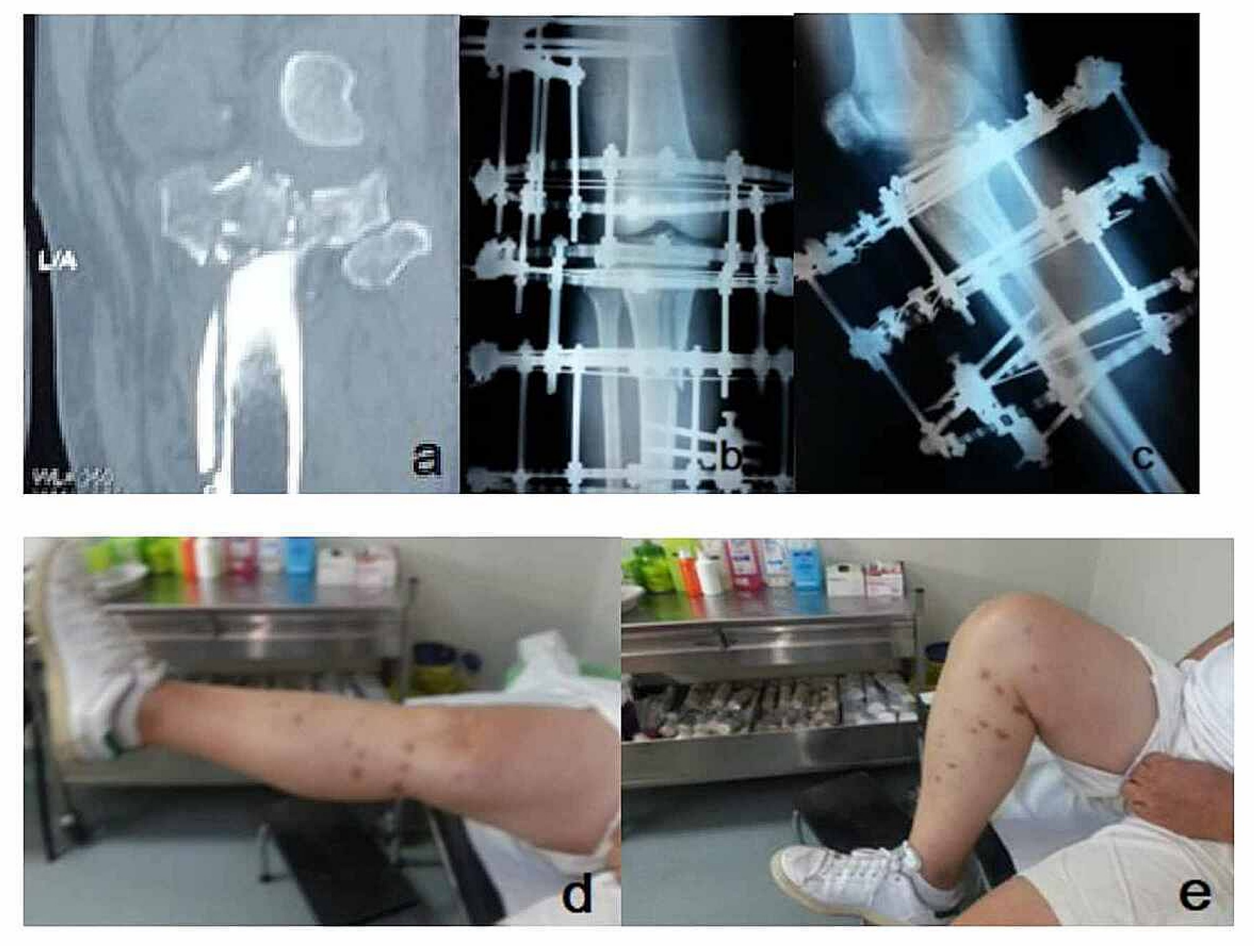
A 65-year-old man with a Schatzker type VI tibial plateau fracture (a) Axial CT scan view of the fracture. (b) An anteroposterior intraoperative radiograph following mini-open reduction of the articular surface and application of an Ilizarov frame with knee bridging. (c) Lateral view of the knee joint after the removal of the knee bridging. (d, e) Range of motion in extension and flexion six months postoperatively in the same patient.
